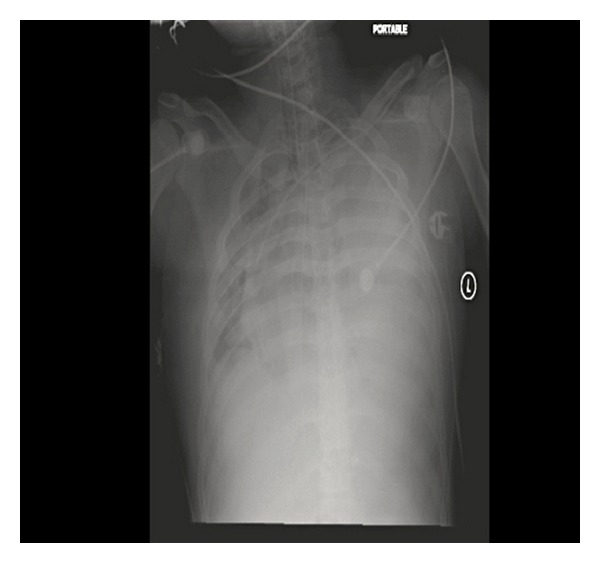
Chest X-ray 5 h after surgery demonstrates complete opacification of the left hemothorax with shift of the mediastinum to the right.
